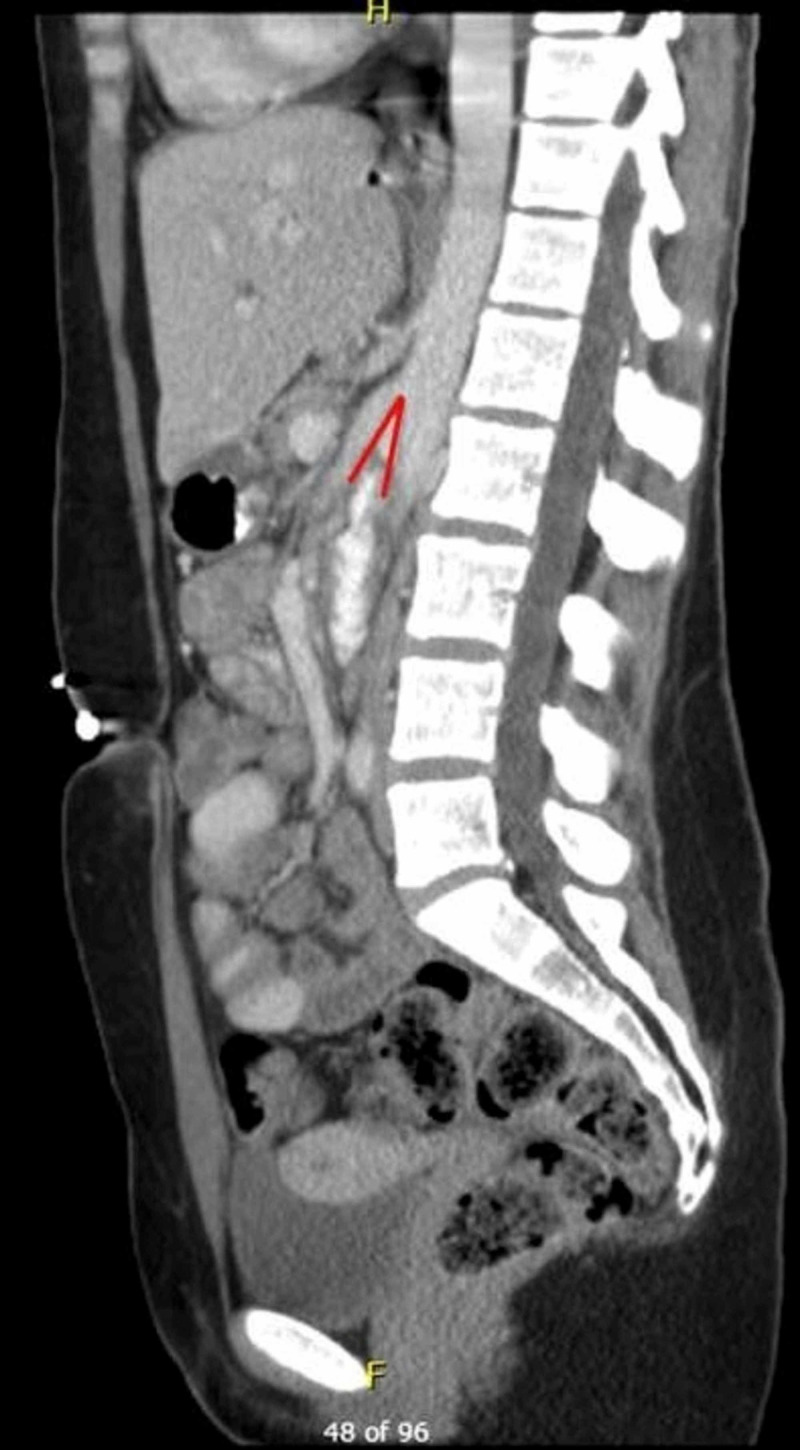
Sagittal computed tomography images reveal transverse duodenum compressed between the aorta and superior mesenteric artery The red bars reveal the aortosuperior mesenteric angle and demonstrate the aortomesenteric distance
